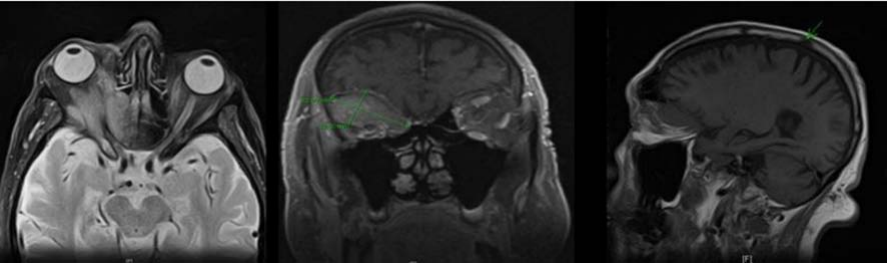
MRI of the head showing a right intra-orbital mass measuring 4.3 × 2.3 cm, extending into the anterior cranial fossa (superiorly); lamina papyracea, posterior ethmoid sinus and anterior lateral sphenoid sinus (medially); and posterior lateral orbital wall and sphenoid wing (laterally); sclerotic lesion in the right parietal bone also noted.
